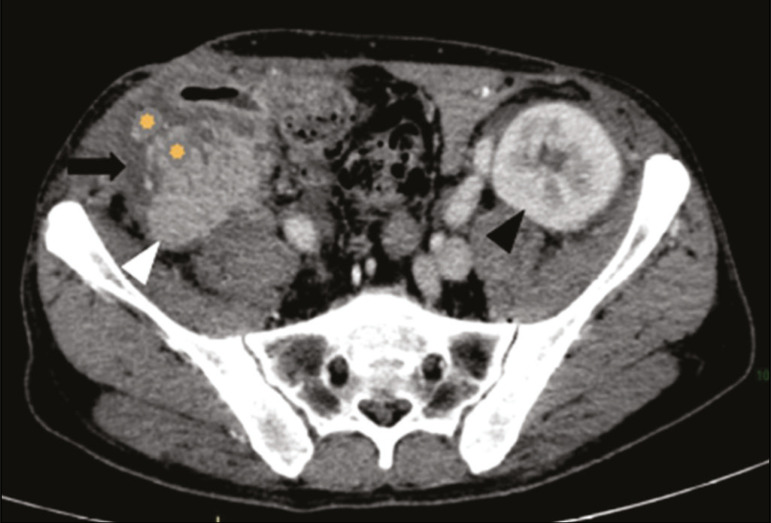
Contrast-enhanced axial CT scan, in the portal phase, of a patient in the postoperative period after simultaneous transplantation of a pancreas (white arrowhead) and a kidney (black arrowhead), presenting a small amount of peripancreatic fluid (arrow), in addition to adjacent lymph nodes (asterisks), usually without clinical significance, which may occasionally mimic some abnormality.
